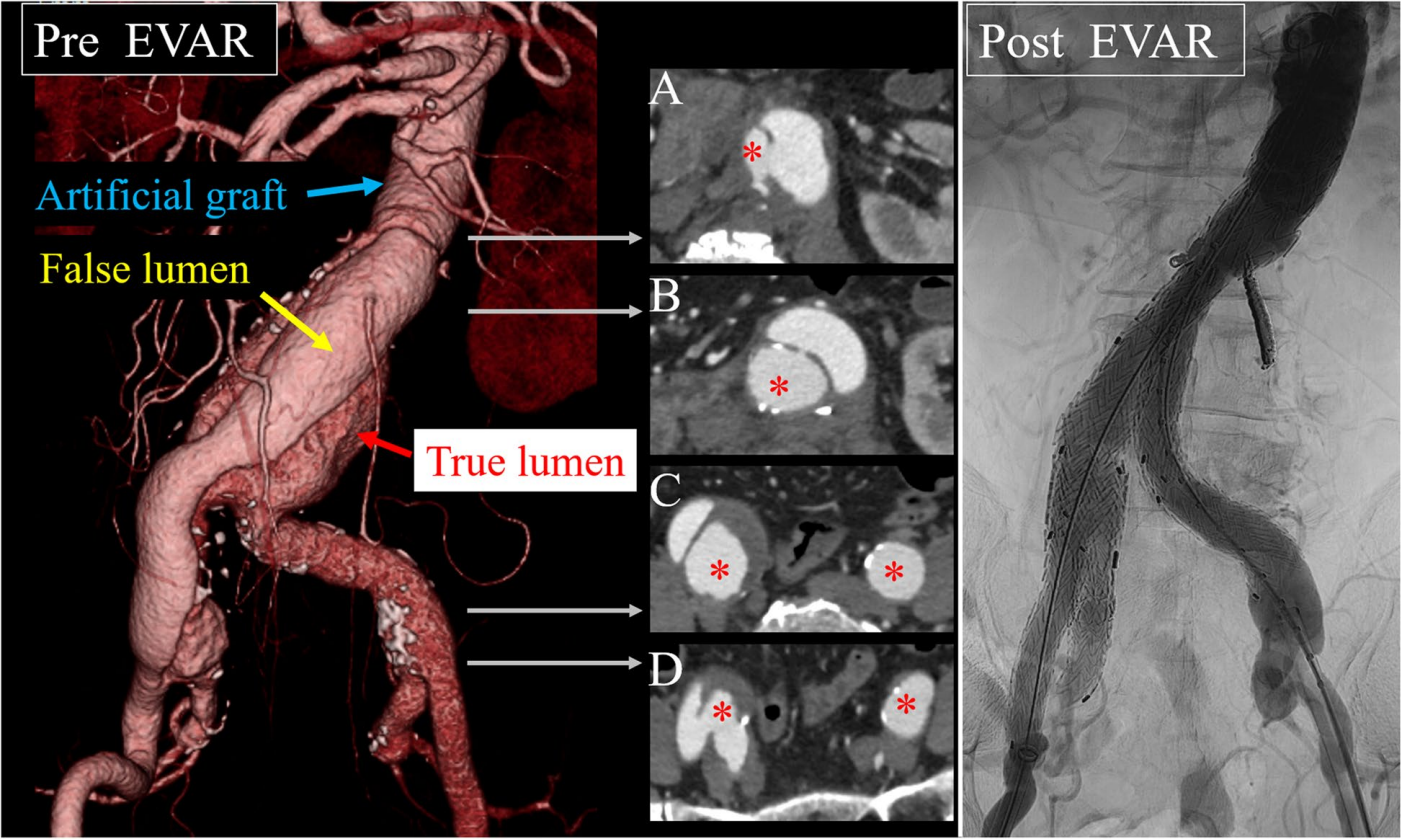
Pre-EVAR-contrast-enhanced CT and post-EVAR-angiography. A Distal surgical graft with a double-barrel anastomosis below the renal artery and a large entry at the same site. B The inferior mesenteric artery branches from the false lumen. C, D Re-entry at the right iliac artery bifurcation and termination of the dissection. *: indicate true lumen. Contrast immediately after EVAR. Angiography reveals no obvious endoleak. EVAR, endovascular aortic repair; CT, computed tomography
